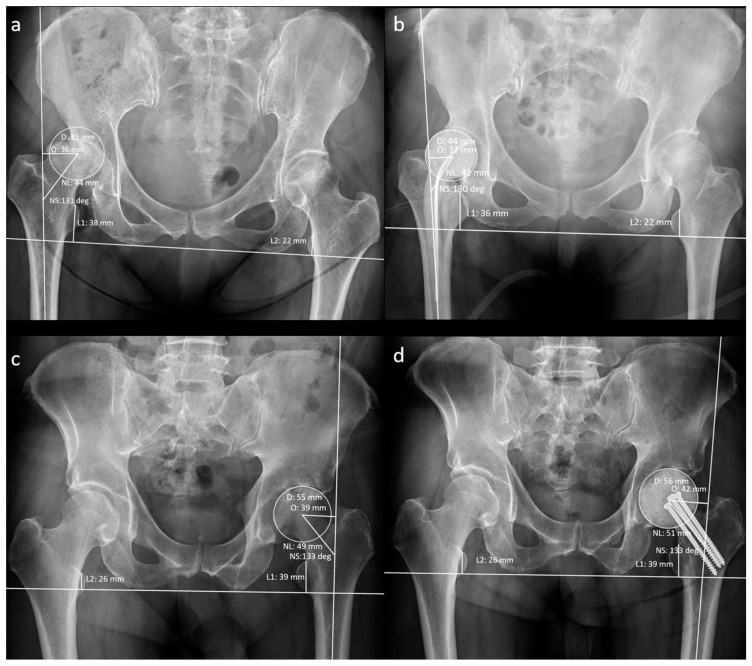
Measurement of radiographic hip geometrical parameters. (a) Pre-operative n-MS hip, (b) post-operative n-MS hip, (c) pre-operative MS hip, and (d) post-operative MS hip. Δhip parameters: femoral head diameter difference (post-operative D–pre-operative D), femoral neck length difference (post-operative NL–pre-operative NL), neck–shaft angle difference (post-operative NS–preoperative NS), femoral offset difference (post-operative O–pre-operative O), and leg-length discrepancy difference (post-operative L1–L2)–(pre-operative L1–L2).
